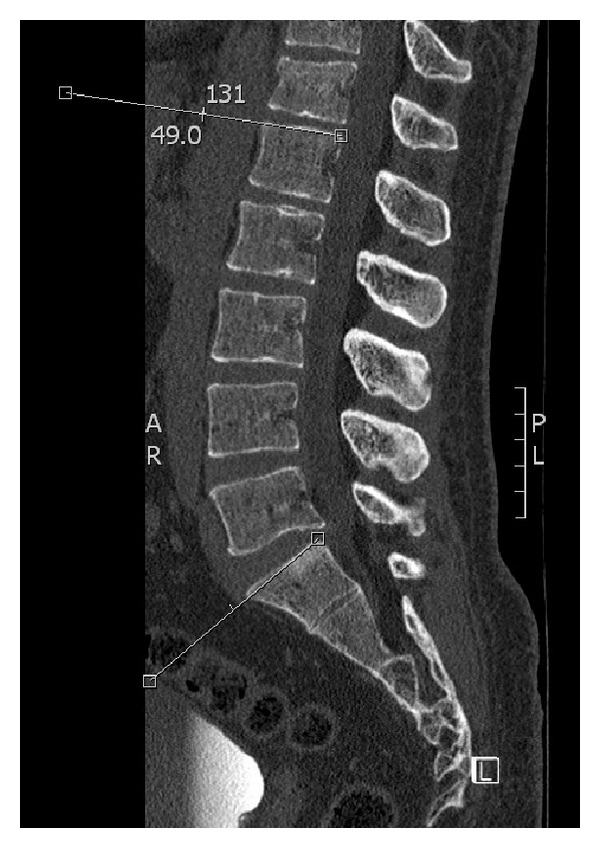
Anatomical Cobb angle measurement by CT.
